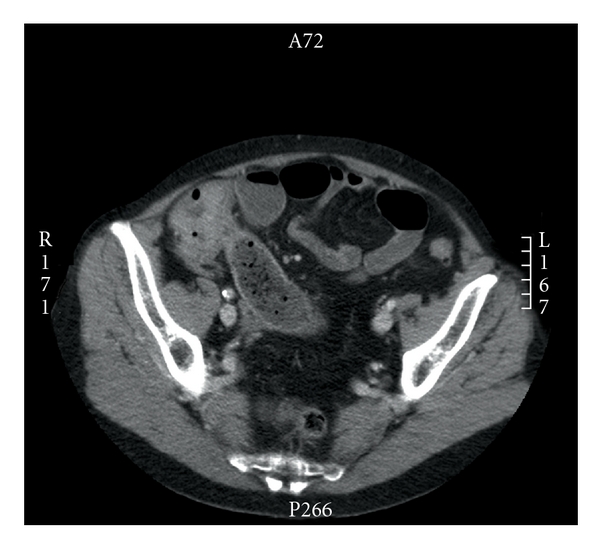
CT transversal image showing a tumor of the colon ascendens.
